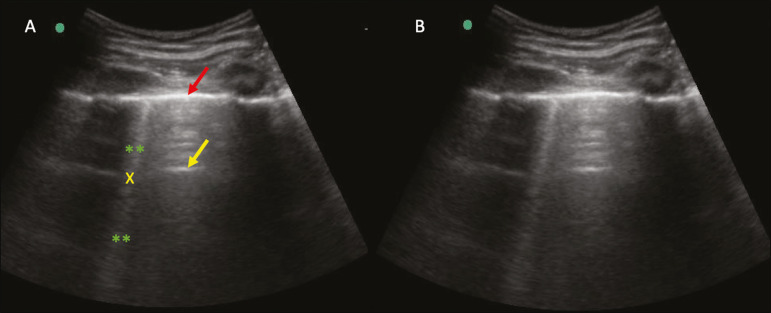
B-mode chest ultrasound. A: Representation with markings corresponding to the A, B, and pleural lines. The B-line (asterisks) represents vertical, hyperechogenic artifacts that cover the entire anteroposterior field analyzed. An important characteristic is that they eliminate the A-lines (yellow arrow) when crossing (X). The pleural line is shown as a hyperechogenic line (red arrow). B: Image demonstrates B-mode chest ultrasound without indicative markings.
